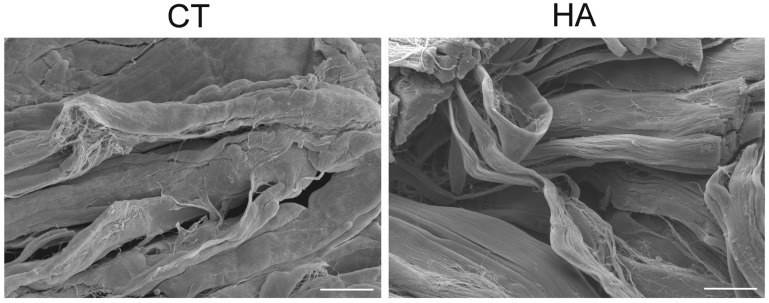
SEM images of reticular dermis in control (CT) and hyaluronic acid-treated (HA) skin at 24 h. Note the looser arrangement and the tortuous pattern of collagen in HA. Bars, 20 µm.
